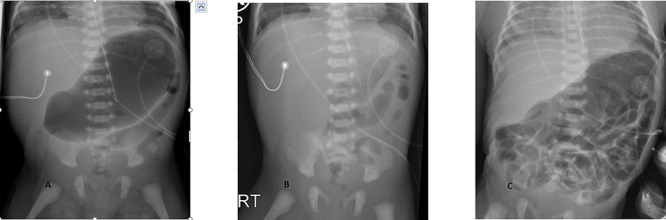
(a–c) Progression of the abdominal X-ray from an appearance of gastric outlet obstruction (a) to fairly decompressed (b) to a complete pattern of distension throughout the stomach, small and large bowels (c).
